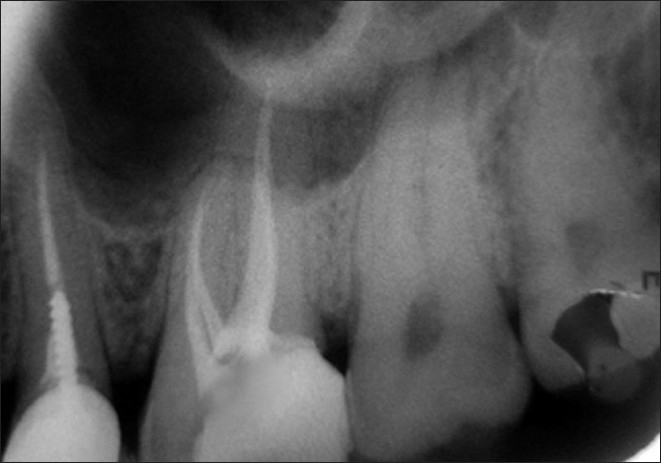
Intra oral radiograph showing obturation of the four canals in relation to #14
